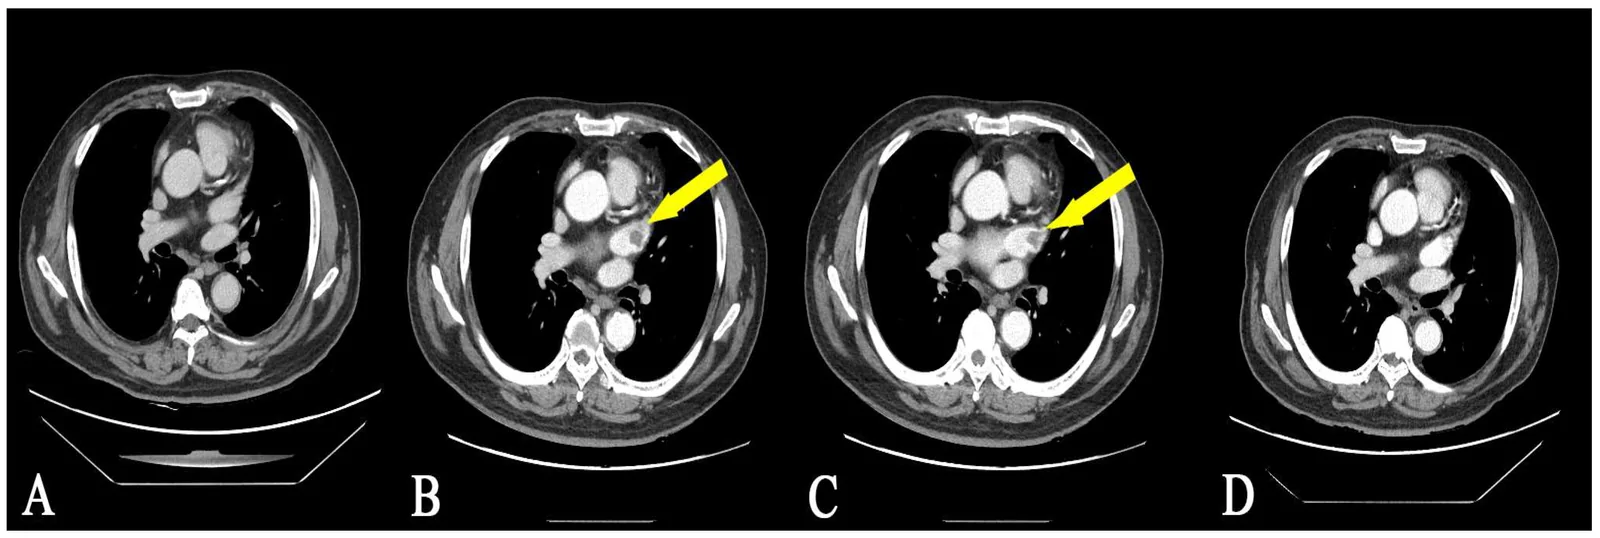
Serial contrast-enhanced computed tomography images of the left atrial appendage obtained from abdominal CT examinations. (A) Day 2. No filling defect is identified in the left atrial appendage on CT. (B,C) Day 10. A 15-mm filling defect is visible in the left atrial appendage (arrows), reported as possible embolic material and radiologically suggestive of thrombus. (D) Day 16. The previously identified filling defect is no longer visible.
